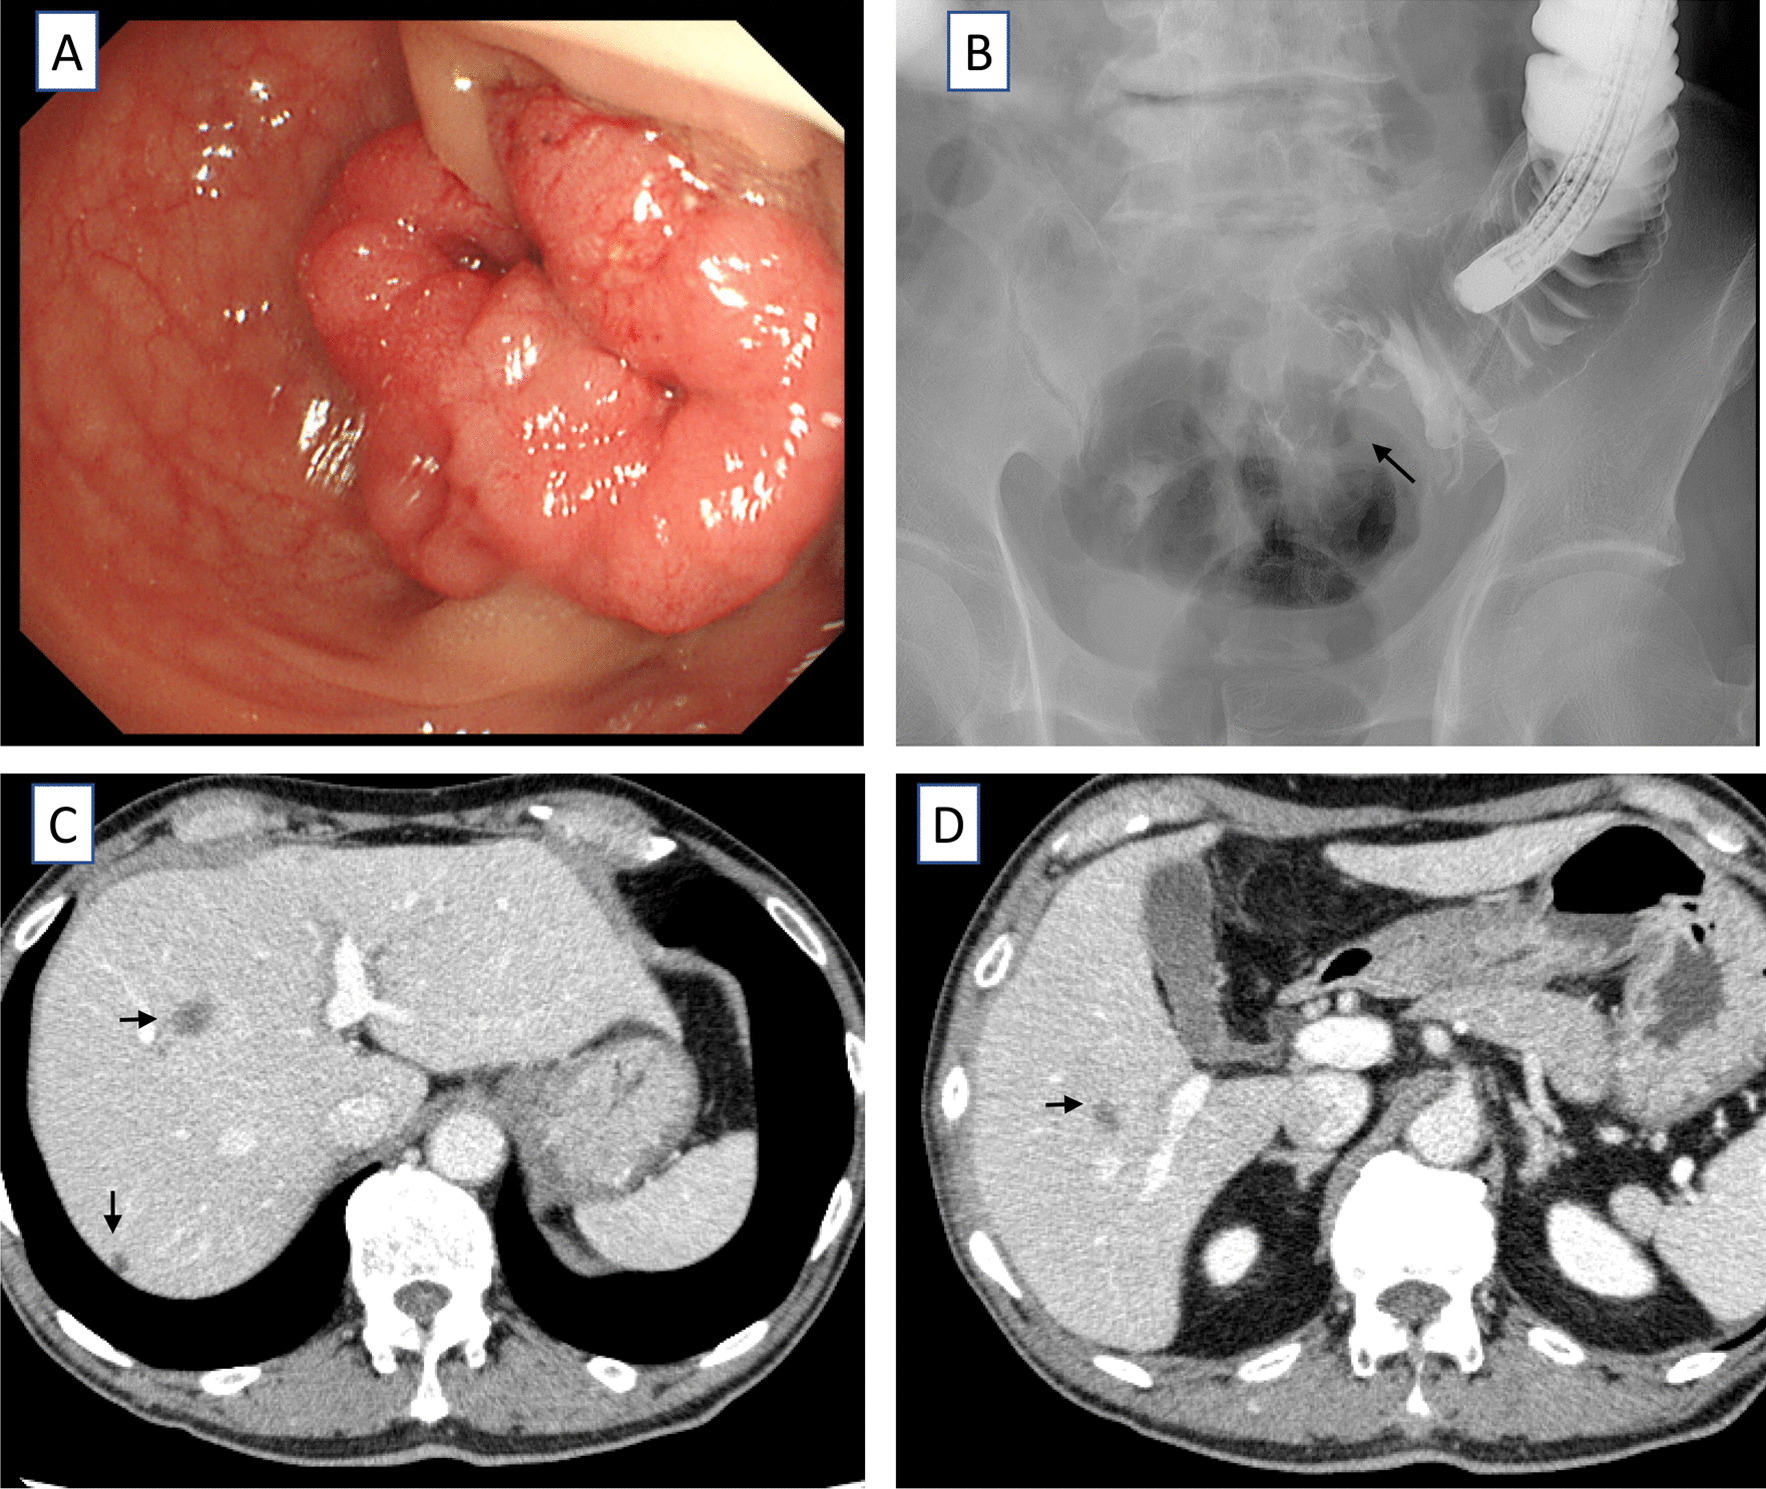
Imaging studies before portal venous embolism was suggested. A Colonoscopy showing the circumferential sigmoid colon cancer. B Findings of barium enema showing an apple core sign. C, D Enhanced computed tomography (CT) showing S8, S7, and S5 metastatic tumors
